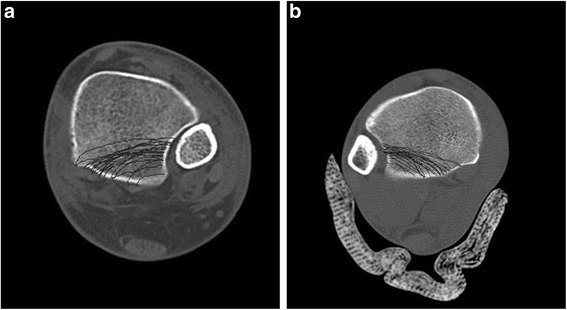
Axial CT (a right side, b left side) scans demonstrating the posterior malleolus fracture pattern. The Posterior Malleolar Map revealed a fracture pattern and morphology consistent with the fracture lines, as originally described by Haraguchi
